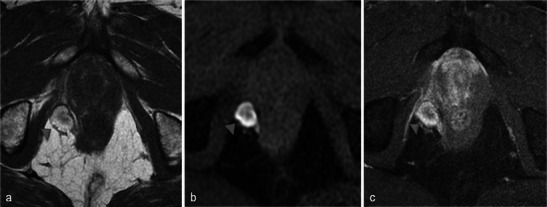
Periprostatic fatty tissue. A 62-year-old patient who had underwent left photodynamic therapy 1 year earlier, and right treatment 7 days earlier. Axial T1-weighted (a), axial T1 FS unenhanced (b) and contrast-enhanced (c) images show a nodular lesion (*arrowhead*) located in the right rectoprostatic angle, with a high intensity T1 FS signal, unenhanced, corresponding to focal haemorrhagic necrosis of periprostatic fatty tissue
